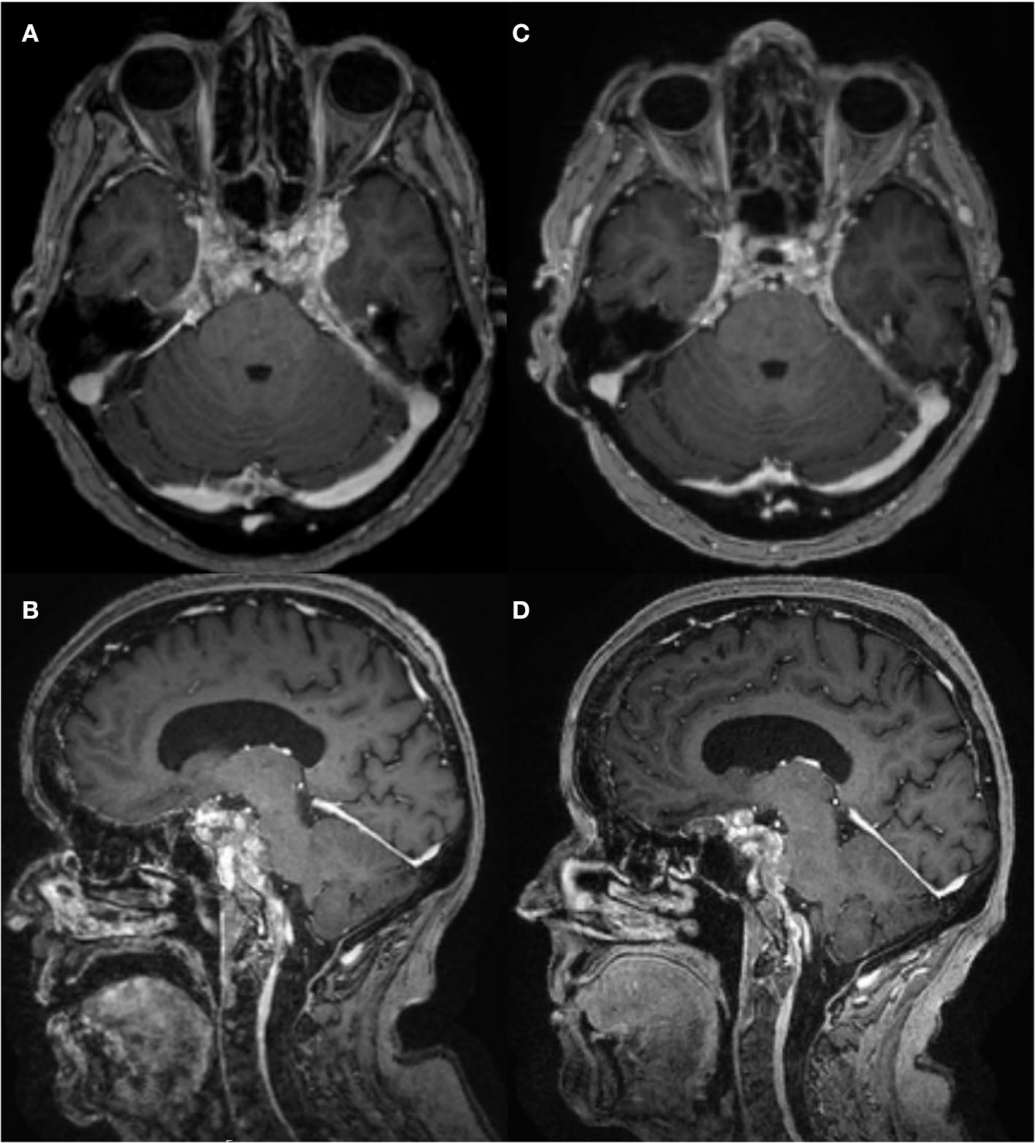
Brain MRI T1 post-contrast, pre- and post-intrathecal rituximab. (A, B) January 2021, before intrathecal rituximab. (C, D) March 2022, after two cycles of intrathecal rituximab. There was mild improvement in enhancement and pachymeningeal bulk after cycle 1, but not after cycle 2.
